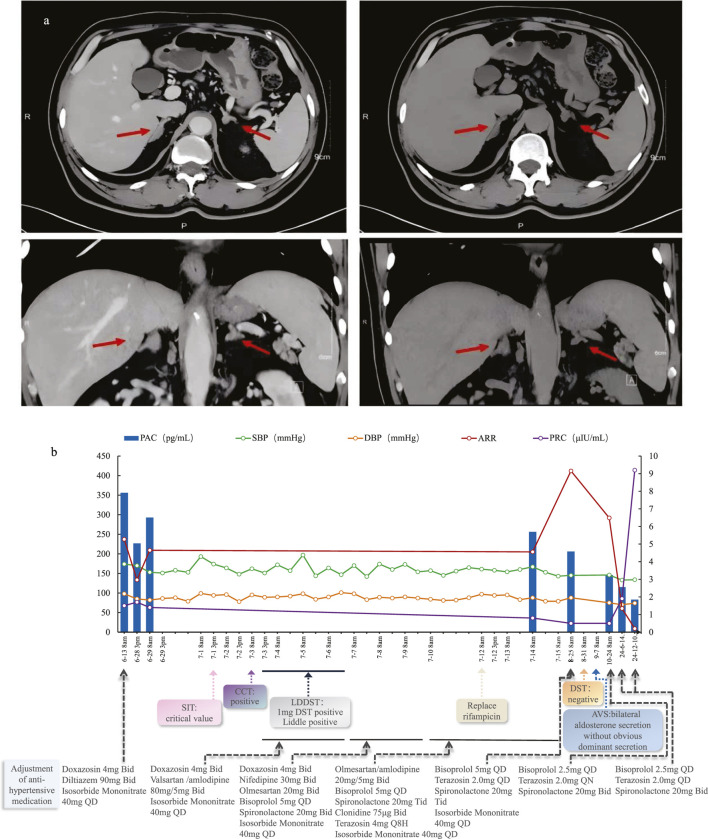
(a) Axial and Coronal adrenal contrast-enhanced CT of this patient; (b) Patient’s diagnostic and treatment process chart.
